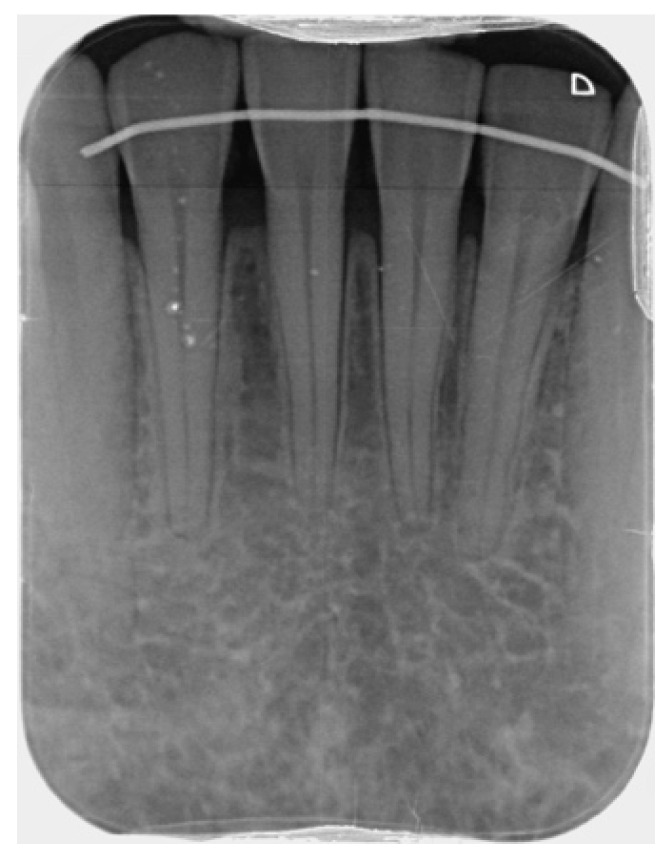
Intra-oral radiograph of the lower incisors.
